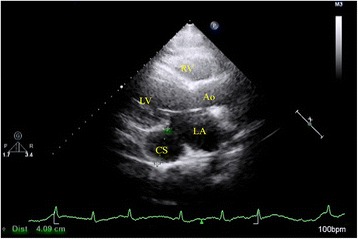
Parasternal long-axis view from echocardiography demonstrated the coronary sinus aneurysm with a diameter of 4.09 cm. Ao: aorta; CS: coronary sinus; LA: left atrium; LV: left ventricle; RV: right ventricle
